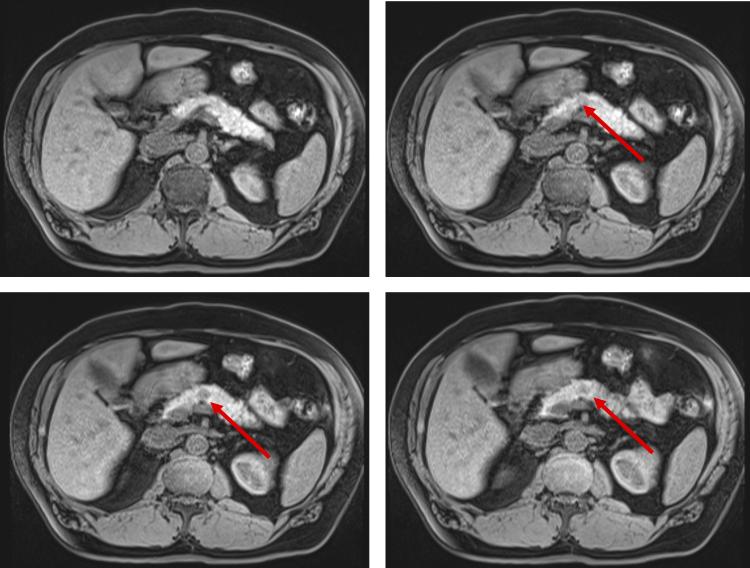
Magnetic resonance imaging of the pancreatic lesion. Red arrows denote the pancreatic lesion.
